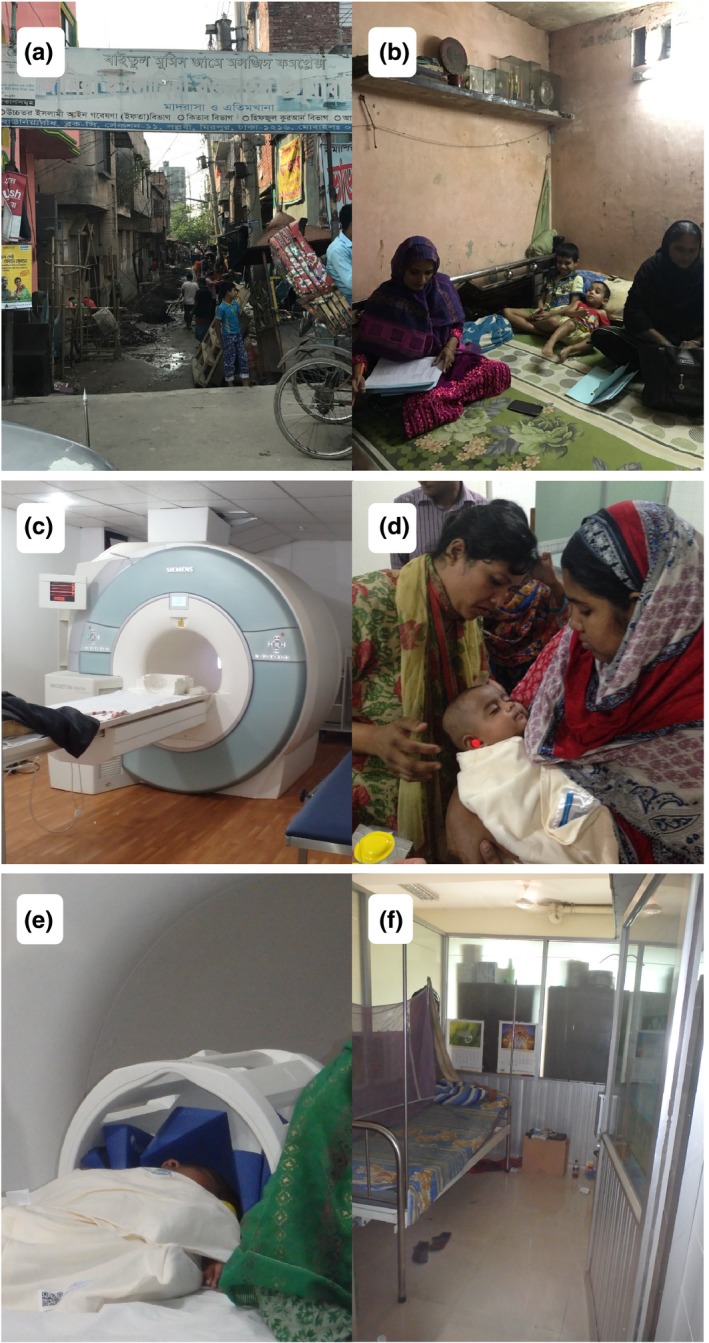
Location of the study. Representative (a) neighborhood and (b) home in Dahka, Bangladesh, where infants in this study reside. (c) MRI facility where infants are scanned. (d) Asleep infant receiving two layers of ear protection. (e) Infant head under head‐coil. As demonstrated, head pads did not tightly secure the infant's head. (f) System administrator's office, used for mothers to put infants to sleep and for final screening
